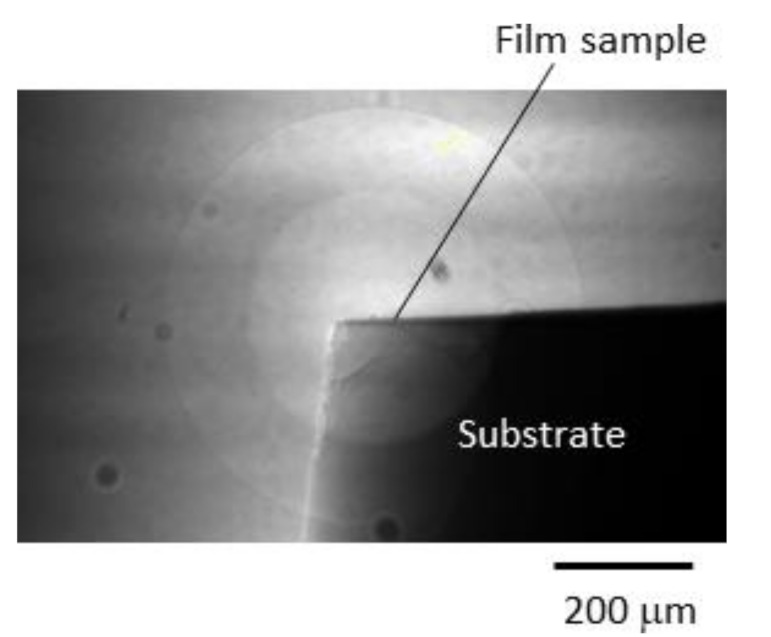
Radiograph obtained by the 2D X-ray detector.
